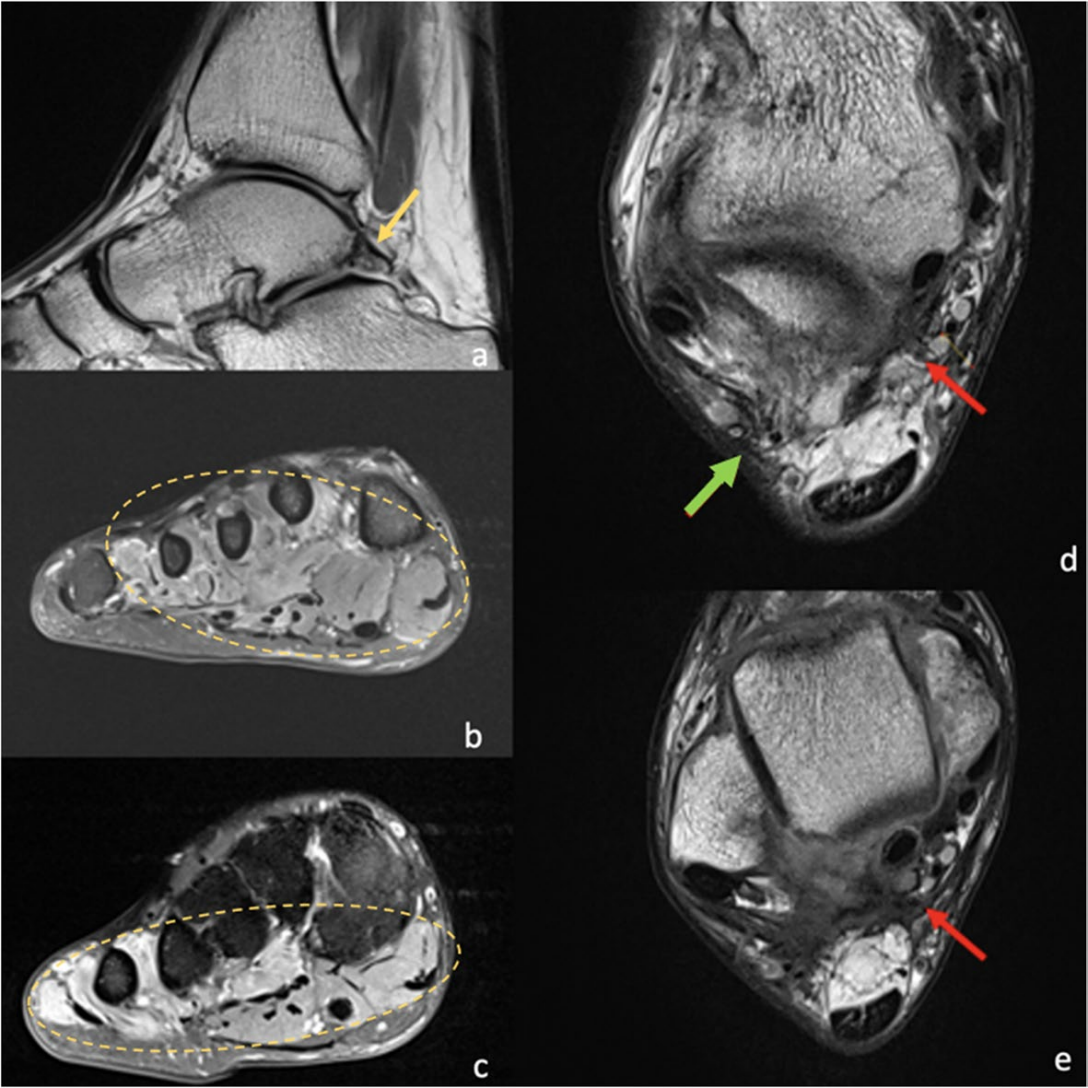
24-year-old male with posterior impingement secondary to an os trigonum, who underwent os trigonum resection. Preoperative sagittal T1 (a) demonstrates the prominent os trigonum (yellow arrow). Post-surgical MRI was performed due to altered sensation in the plantar forefoot laterally. Post-surgical MRI in the short axis PDFS (b and c) sequences demonstrates extensive muscle oedema and atrophy (circled) involving the lateral lumbricals, abductor digiti minimi, quadratus plantae, adductor hallucis and flexor digiti minimi brevis consistent with lateral plantar nerve impingement. Axial PD (d and e) sequences demonstrate adhesions and granulation tissue formation, extending towards the sural nerve posterolaterally (green arrow) and lateral plantar nerve medially (red arrows), resulting in impingement of the nerves
